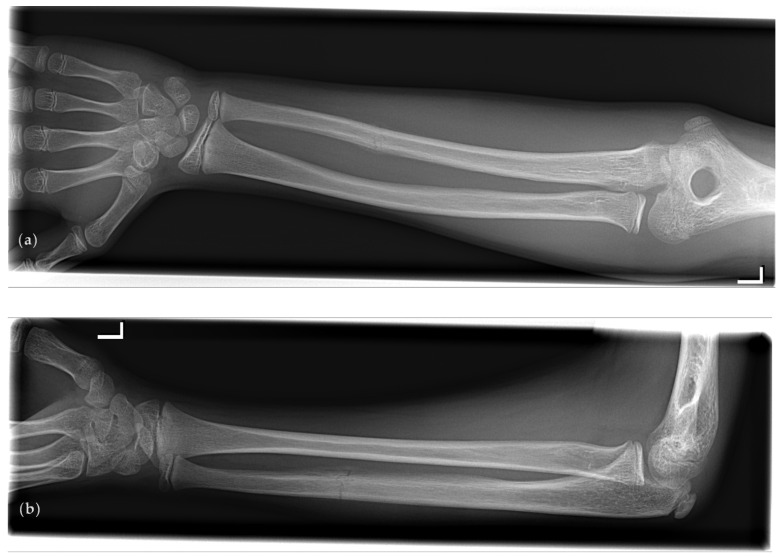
A typical example of an isolated fracture of the left ulna that meets all four parry fracture criteria according to Judd [1]: absence of radial involvement, transverse fracture line, distal from midshaft, and minimal displacement. Radiographs are shown in (a) anteroposterior projection; (b) lateral projection.
